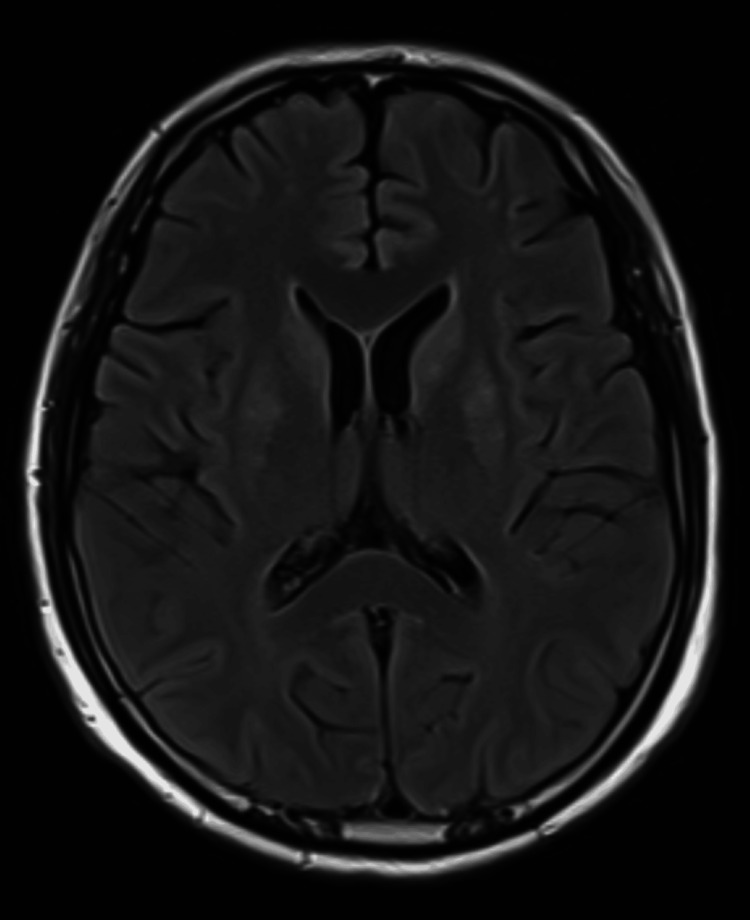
T2-FLAIR-weighted cranial MRI excluded hypoxic-ischemic lesions secondary to cardiorespiratory arrest FLAIR: fluid-attenuated inversion recovery; MRI: magnetic resonance imaging
